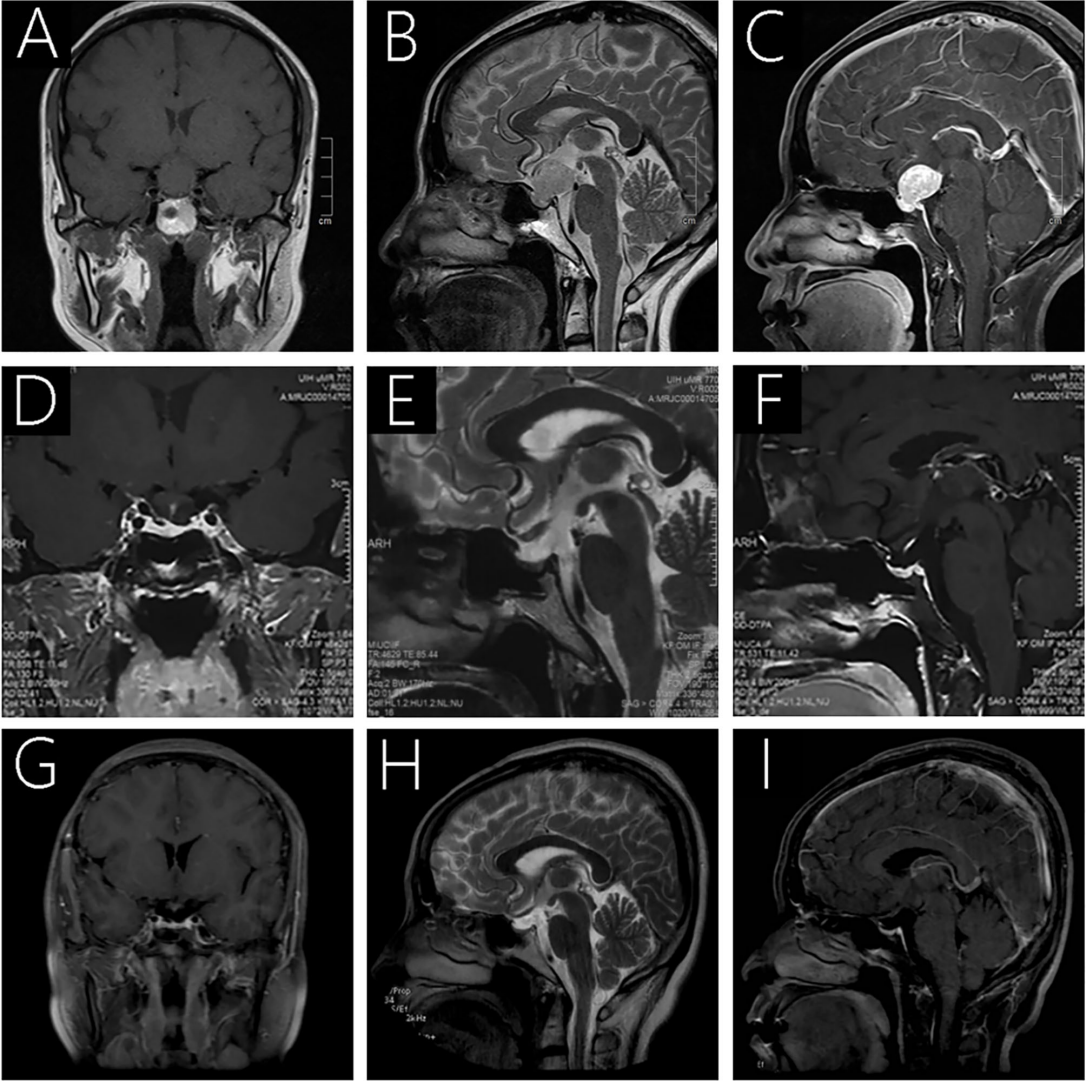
Preoperative MRI (A-C) revealed a 1.94×1.91×2.16-cm well-defined mass located in the sellar and suprasellar regions. It was isointense on T1WI (A) and slightly hyperintense on T2WI (B), and the tumor was strongly and irregularly contrast-enhanced after gadolinium administration (C). Follow-up MRI 3 months (D–F) and 27 months after surgery (G–I) showed complete removal of the lesion, with no sign of recurrence.
